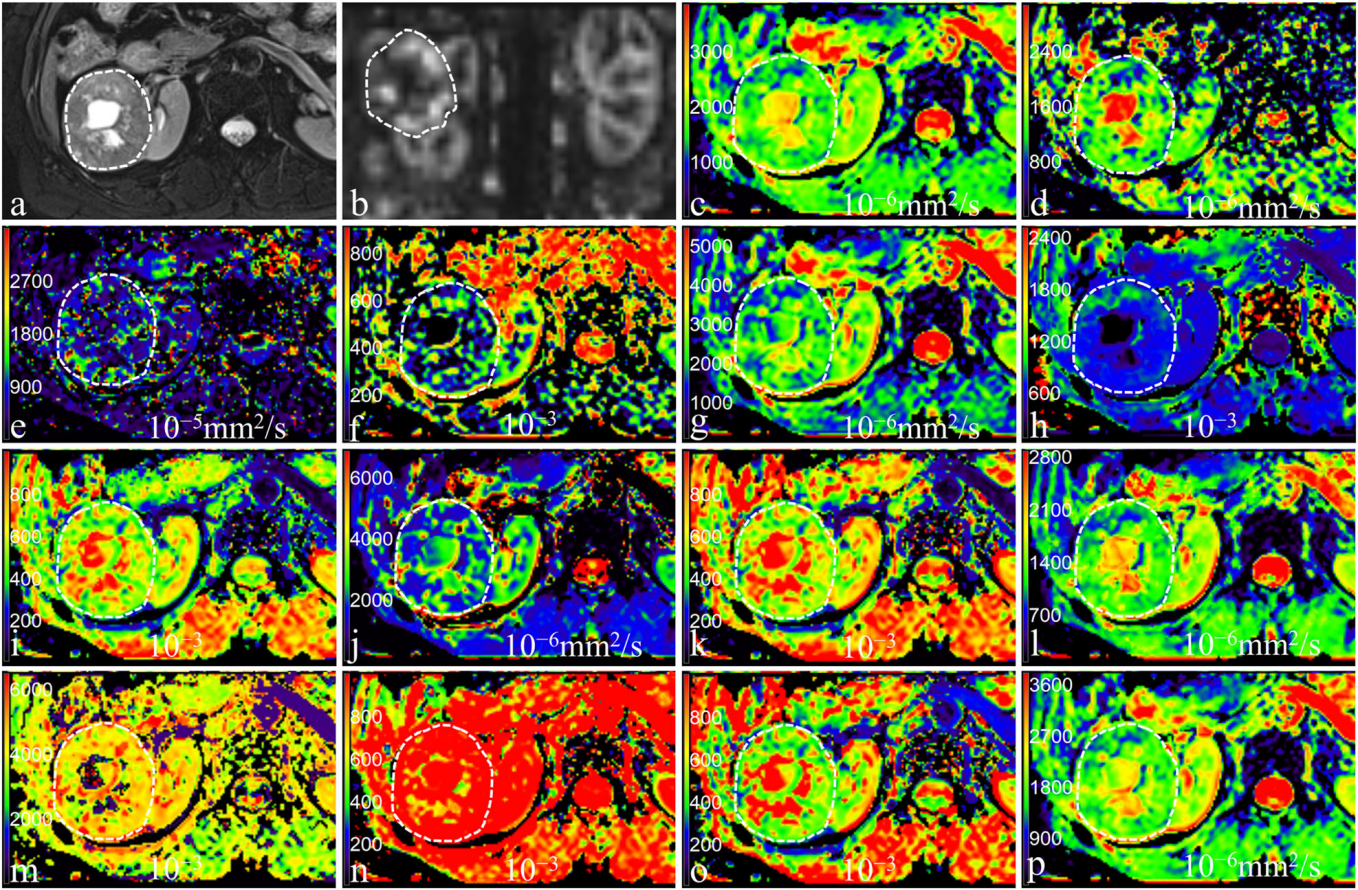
A 61-year-old woman with pathologically confirmed clear cell renal cell carcinoma in the right kidney. a Axial T2-weighted imaging shows a mixed-signal-intensity mass; b RBF map shows the perfusion level of mass, with the RBFmean and RBFpeak of 167.45 mL/100 g/min and 384.00 mL/100 g/min; c–p pseudo-colorized images showed the Mono_ADC (c), IVIM_D (d), IVIM_D* (e), IVIM_f (f), DKI_MD (g), DKI_MK (h), SEM_α (i), SEM_DDC (j), FROC_β (k), FROC_D (l), FROC_μ (m), CTRW_α (n), CTRW_β (o), and CTRW_D (p), with the values of 1.79 × 10^−3^ mm^2^/s, 1.52 × 10^−3^ mm^2^/s, 11.5 × 10^−3^ mm^2^/s, 20.96%, 2.25 × 10^−3^ mm^2^/s, 0.60 × 10^−3^, 0.63 × 10^−3^, 2.36 × 10^−3^ mm^2^/s, 0.67 × 10^−3^, 1.41 × 10^−3^ mm^2^/s, 4.03 × 10^−3^, 0.94 × 10^−3^, 0.64 × 10^−3^, and 1.87 × 10^−3^ mm^2^/s, respectively
